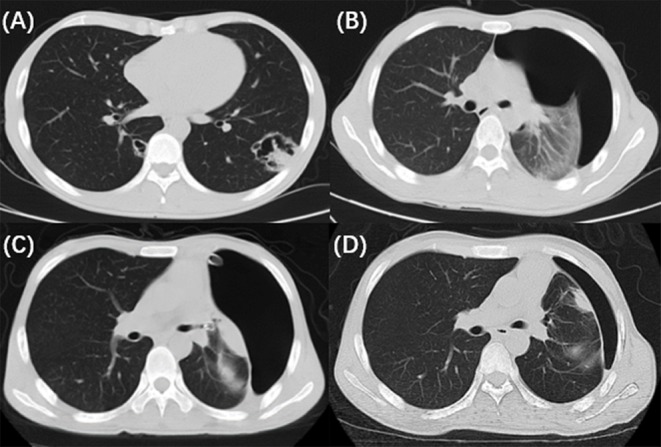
The radiological and bronchoscopic imaging manifestation. (A) Chest CT revealed an irregular thick-walled cavitation in the left lower lobe and cystic lesions in the right lower lobe. (B) Repeat chest CT on the 18th day showed pneumothorax in the left lung. (C) A follow-up chest CT (2 days after EBV implantation) showed partial regression of the pneumothorax. (D) Chest CT acquired after the valves removed revealed that the pneumothorax improved gradually.
